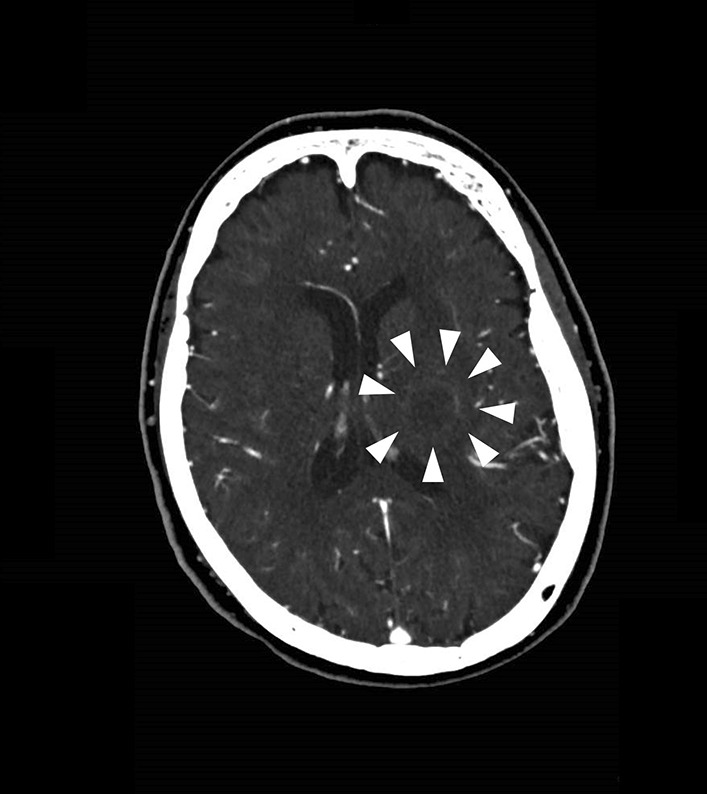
Initial computed tomography of the brain shows low-dense rounded walled lesion measuring 20×16 mm in the left basal ganglia (arrow head)
